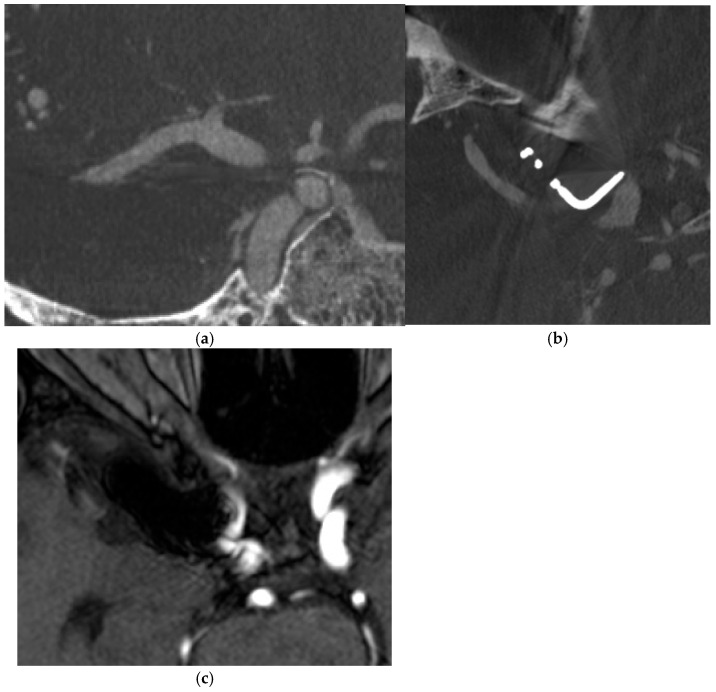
Clipping of right terminal internal carotid artery (ICA) aneurysm. (a,b) IV CBCT with MAR delineated clip well with no residual aneurysm and patent right internal carotid artery (ICA). (c) MRA demonstrates clip leading to marked susceptibility artifacts which render assessment impossible.
